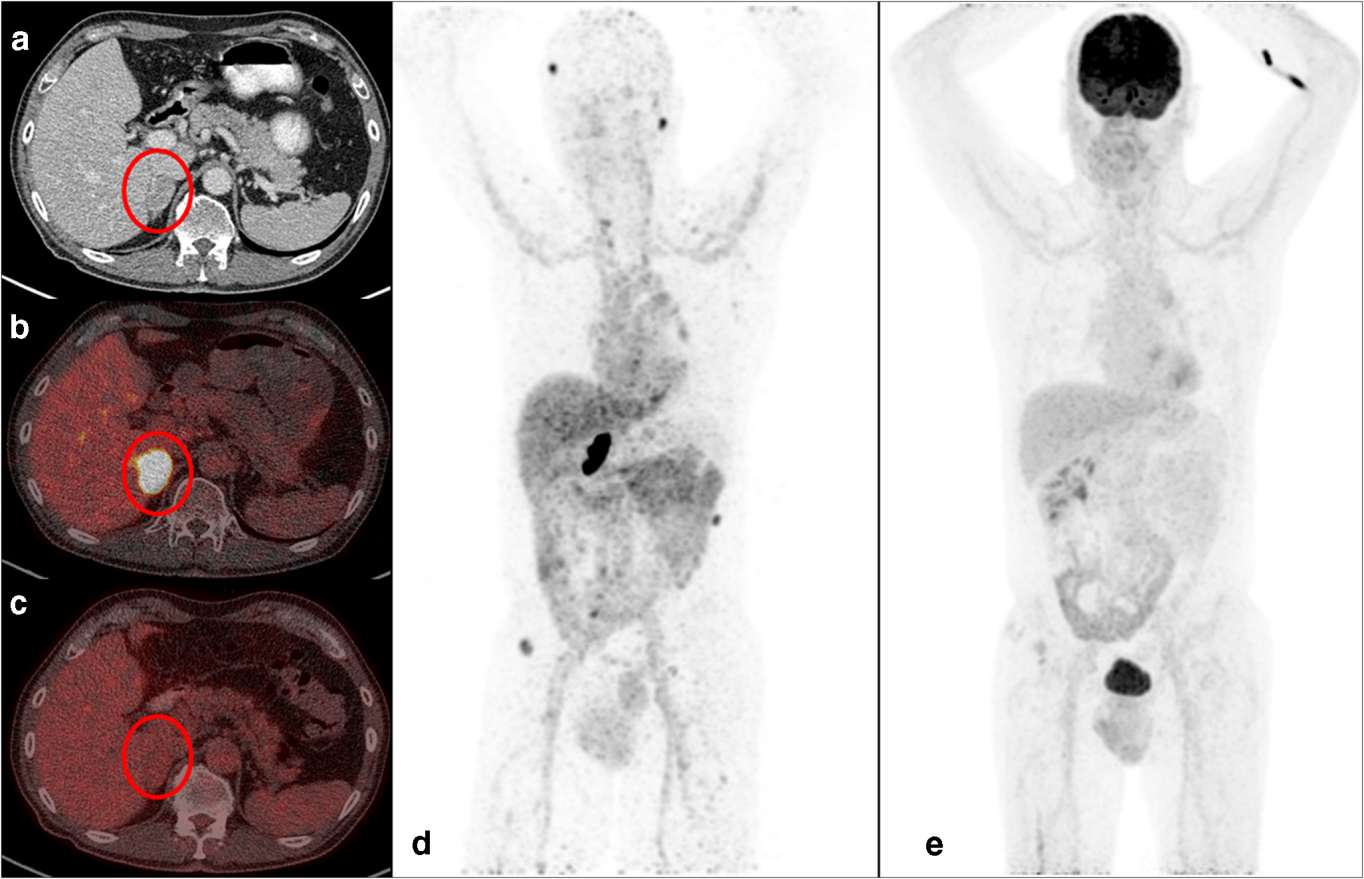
On the left are transversal sections of one patient of CT, [^89^Zr]Zr-DFO-girentuximab and [^18^F]FDG-PET/CT. The *red circle* represents an adrenal gland lesion in a patient as visualized by CT (a), [^89^Zr]Zr-DFO-girentuximab (b) and [^18^F]FDG-PET/CT (c), respectively. On the right, MIP images of [^89^Zr]Zr-DFO-girentuximab (d) and [^18^F]FDG-PET/CT (e) are presented
